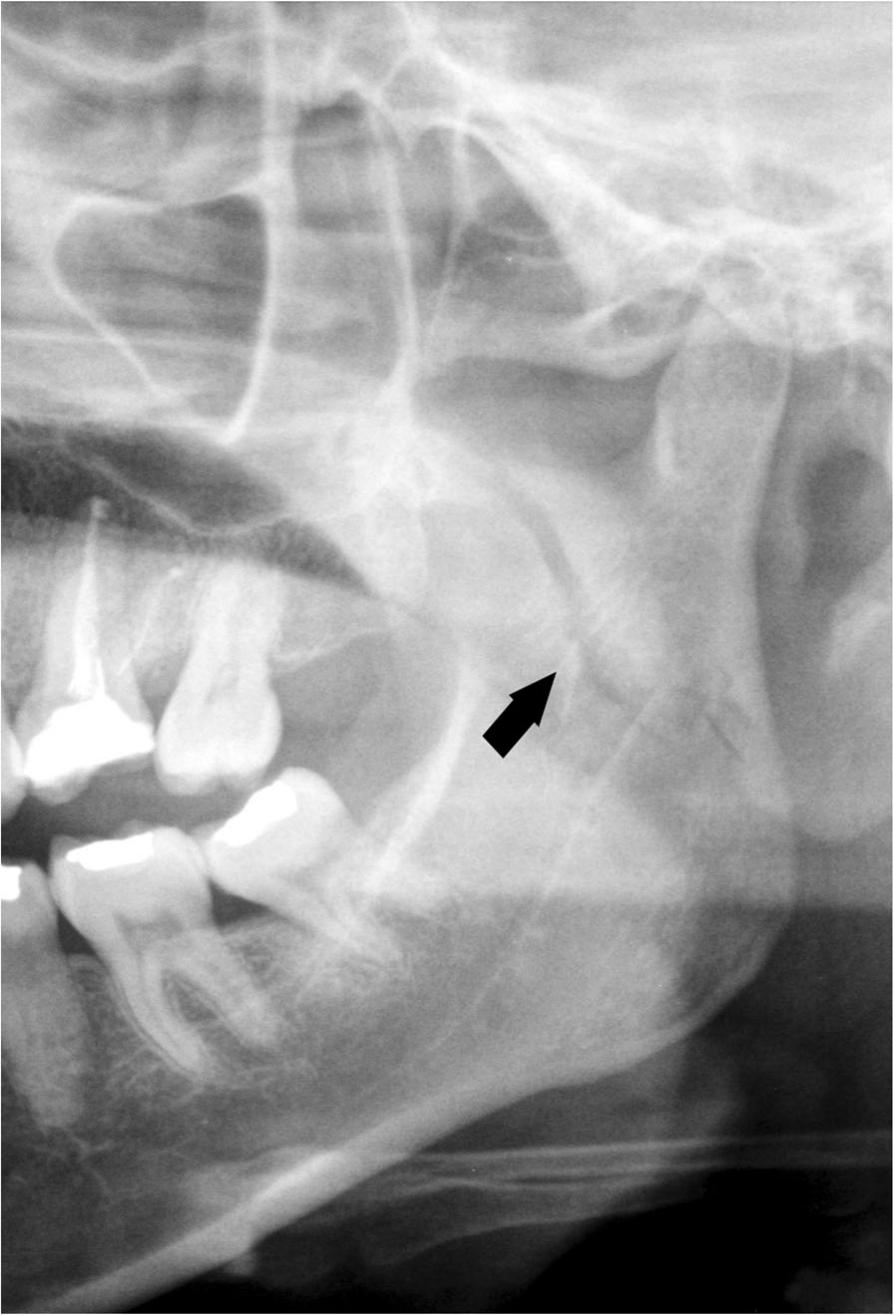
Vertical ramus fracture. Cropped panoramic radiograph showing a fracture of the left mandibular ramus, which runs from the external face of the ramus to the sigmoid notch (arrow)
